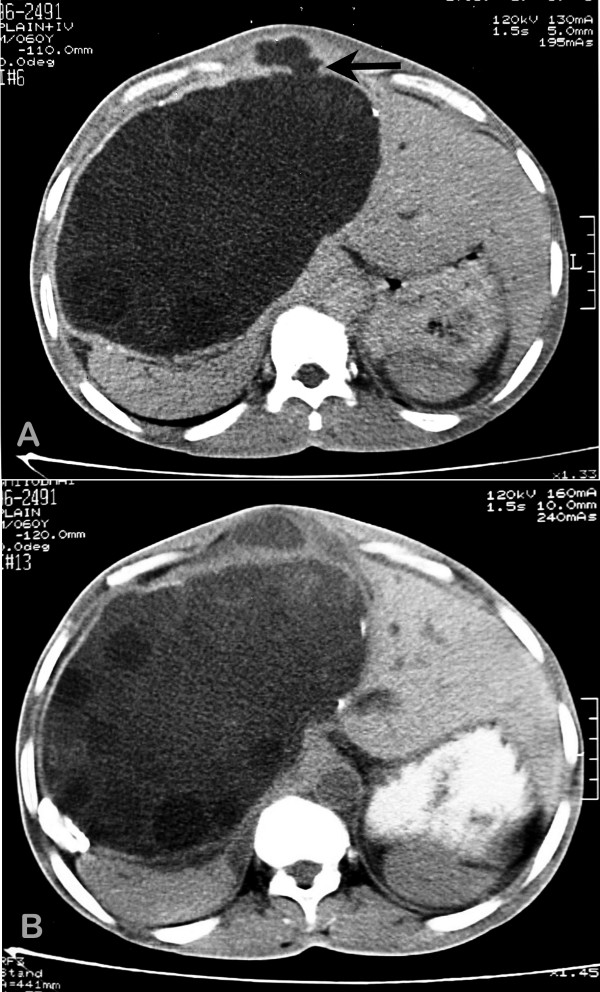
A) & B) Axial spiral CT scan of abdomen through two different levels showing a hydatid cyst in the left lobe (Segments V, VI, VII, VIII) with a cystic projection anteriorly.
